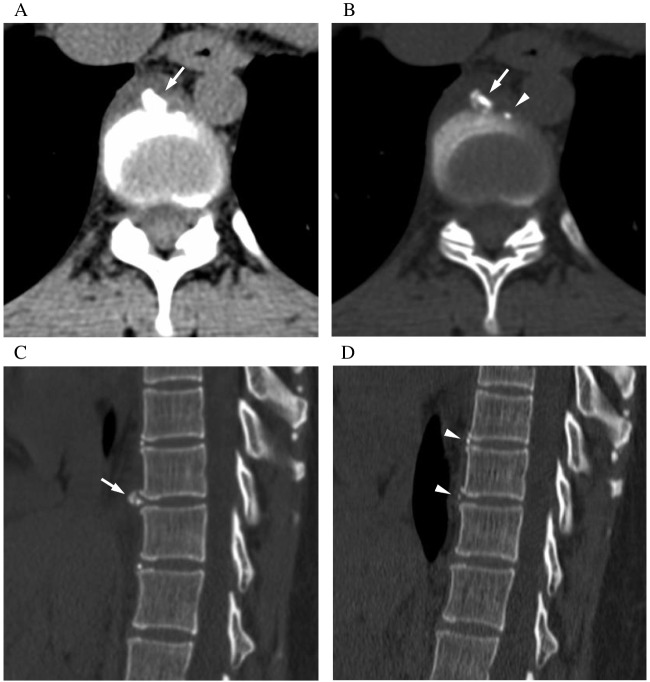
A 37-year-old woman with lower back pain (Patient #2). A Axial CT images at the level of Th10/11 intervertebral disc reveals calcification with soft tissue density at the anterior aspect of vertebrae. B In axial CT images with bone window setting, the calcification appears heterogeneous with slightly hypodense central portion (arrow). On the left side of this lesion, punctate calcification of the intervertebral disc’s annulus fibrosus is also seen (arrowhead). C Sagittal CT image shows paravertebral calcification at the level of Th10/11 adjacent to the calcification of the annulus fibrosus (arrow). D In the sagittal CT image obtained 3 months later, the calcification has resolved. Note that there is no interval change in the intervertebral disc calcification at Th9/10, Th10/11, and Th11/12 (arrowheads)
